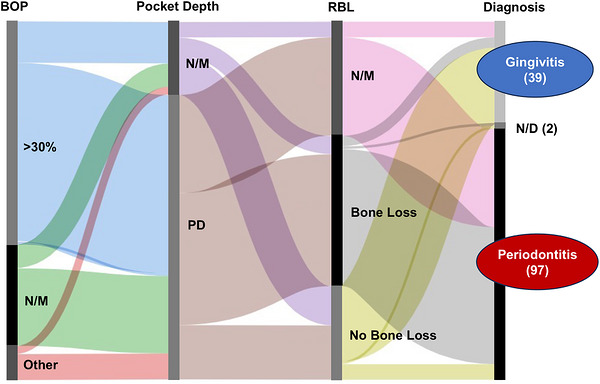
Alluvial plot for periodontal diagnosis (Case 1, Question 1, *N* = 138). BOP, bleeding on probing; N/M = not mentioned; Other included “10‐30% BOP” and “yes”; PD, pocket depth; RBL, radiographic bone loss; N/D, not determined.
